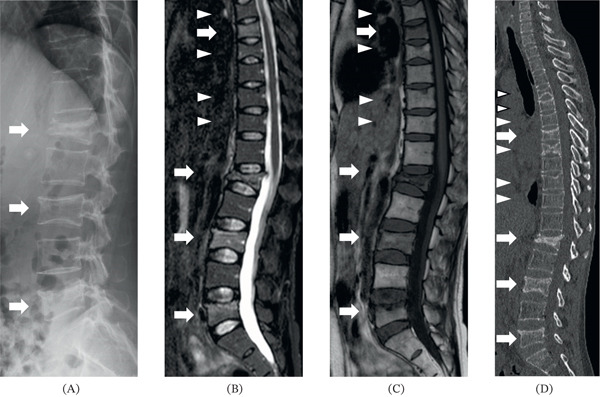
Imaging findings at the clinic visit and during emergency evaluation. (A) Lumbar radiographs obtained at the initial clinic visit showing fractures at L1, L3, and L5 (white arrows). (B) STIR sagittal MRI and (C) T1‐weighted sagittal MRI of the thoracolumbar spine performed after emergency transfer. Acute/subacute fractures with bone marrow edema are seen at T7, L1, L3, and L5 (white arrows) on the STIR sequence, whereas vertebral deformities at T6, T8, T10, and T11 show no marrow edema, consistent with chronic fractures (white arrowheads). (D) Whole‐spine CT (sagittal reconstruction) performed at the emergency hospital showing additional deformities at T4 and T5 (black‐outlined white arrowheads); their acuity could not be determined because these levels were outside the MRI field.
